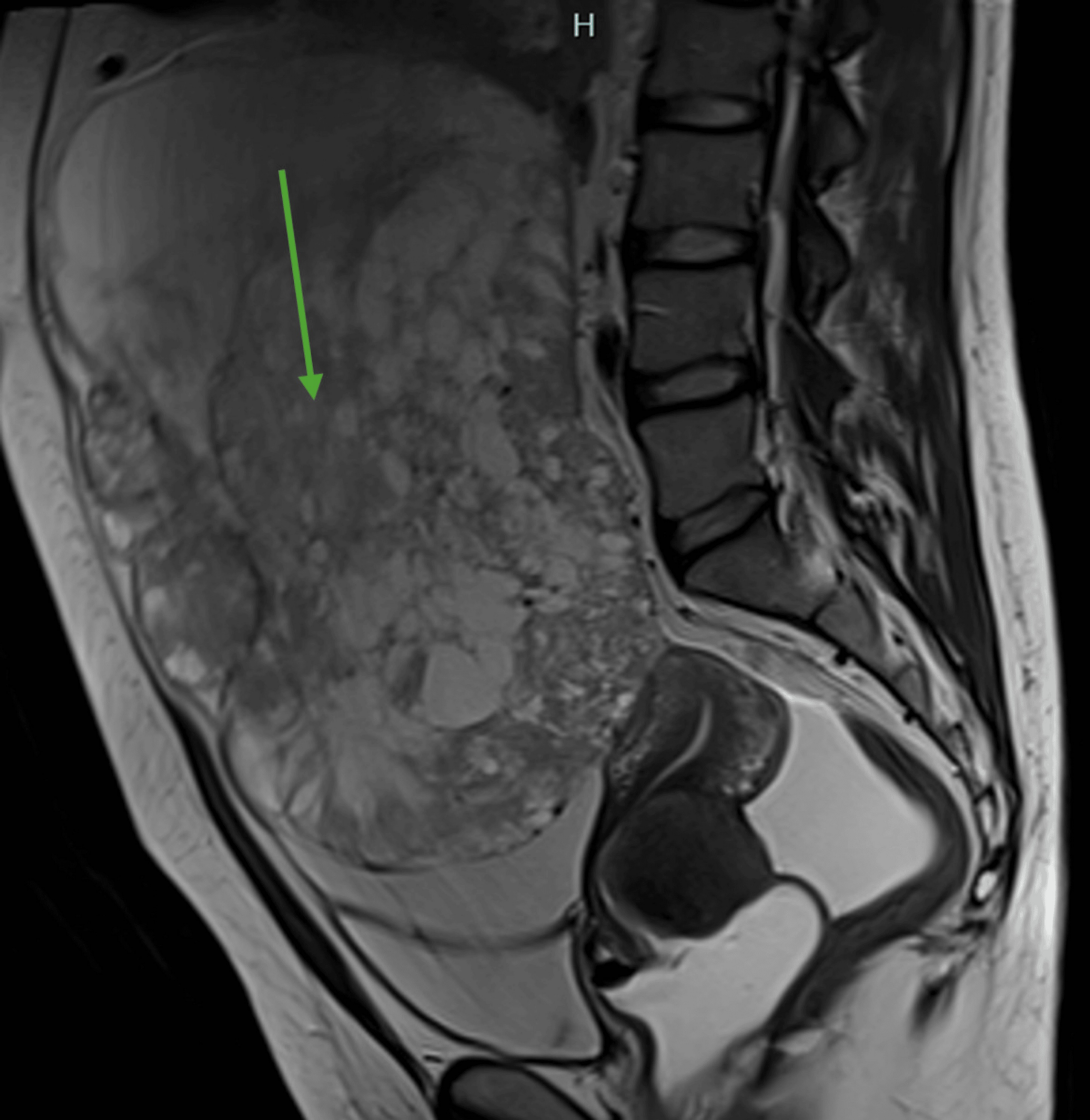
Abdominal MRI This MRI shows disease progression on first-line treatment. The mass previously visible arising from the left ovary (green arrow) now extends far beyond the pelvic cavity and into the abdomen. A cervical mass is also still present.
